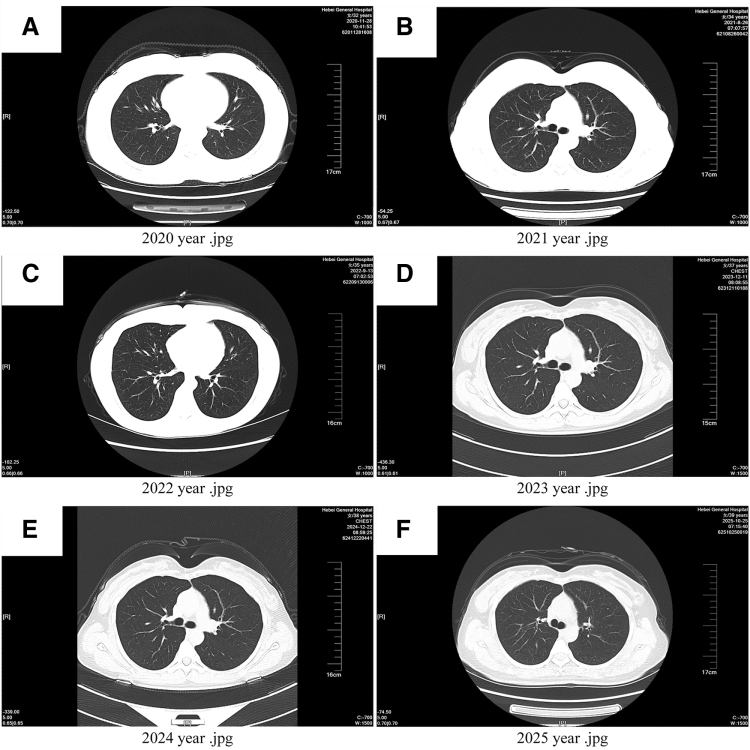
Serial chest CT images of the anti-Jo-1 antibody-positive patient from 2020 to 2025 (A–F). A few stable tiny solid nodules are visible, with no reticular opacities, honeycombing, or consolidation observed.
